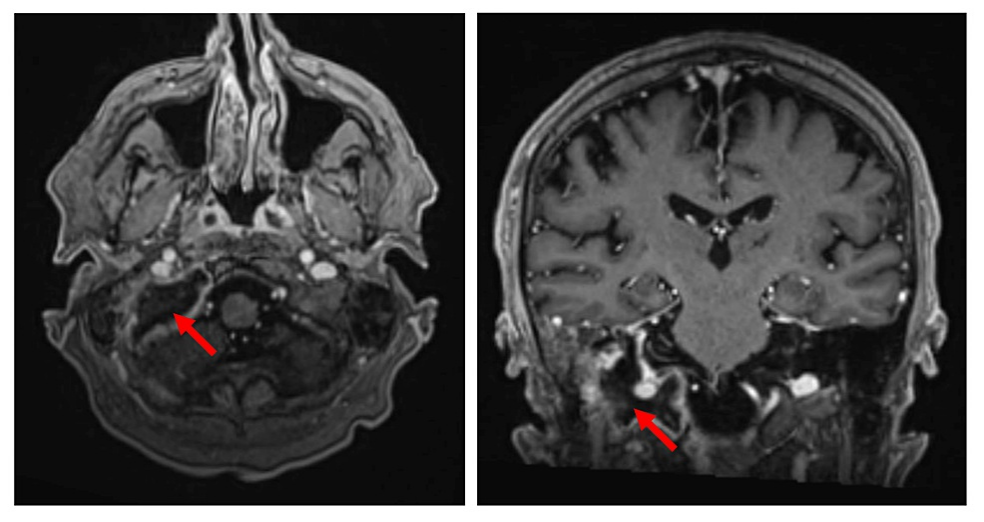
Brain imaging two months after radiotherapy treatment of the jugular foramen plasmacytoma. Axial (left hand side image) and coronal (right hand side image) T1-weighted post-contrast magnetic resonance imaging (MRI) showing complete cystic degeneration of the tumour which is no longer contrast enhancing.
